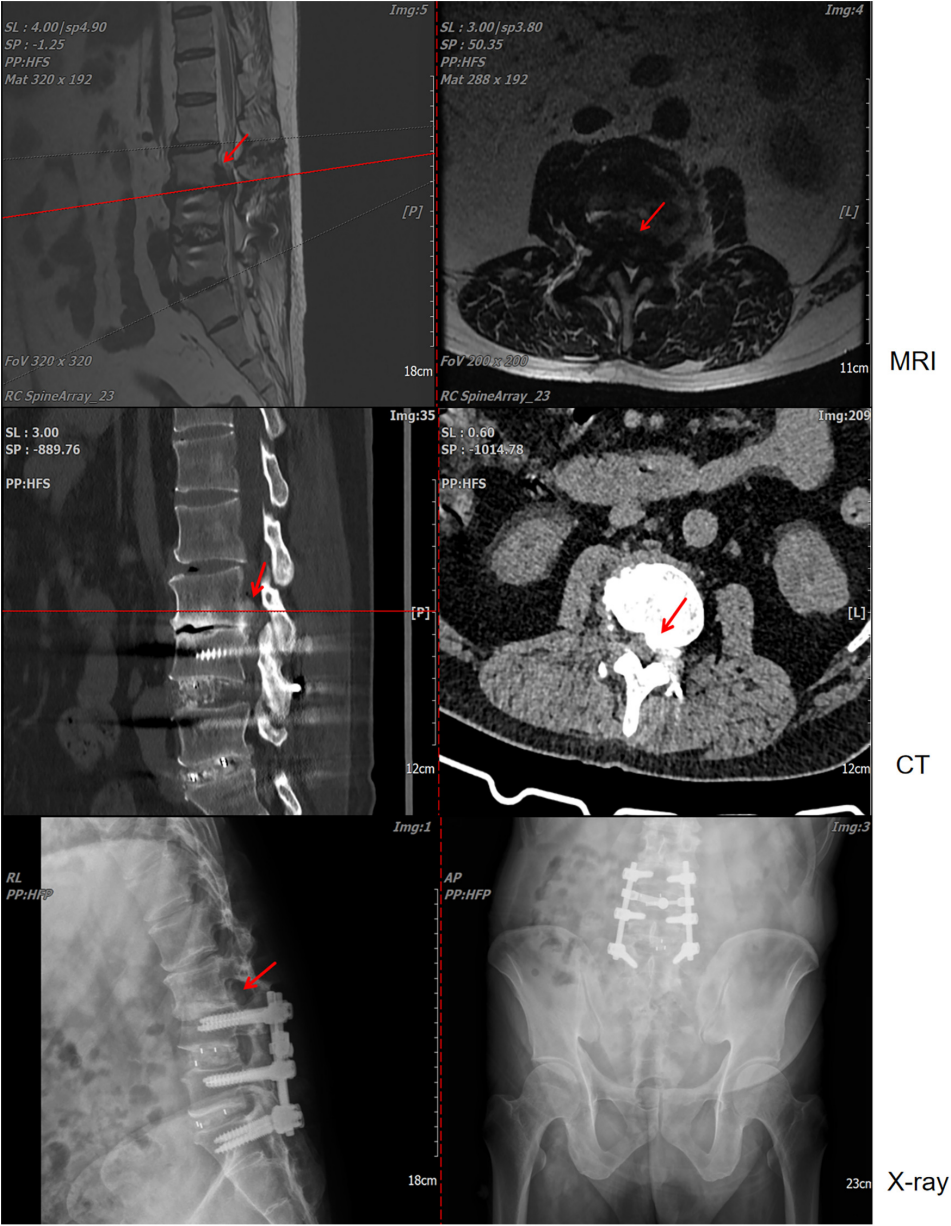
Lumbar magnetic resonance imaging (MRI), computed tomography (CT), and x-ray examinations revealed L4-S1 lumbar fusion with screw rod internal fixation. Concurrently, imaging indicated that the lesion at the posterior edge of the L3 vertebral body exerted compression on the dural sac, resulting in secondary spinal stenosis.
